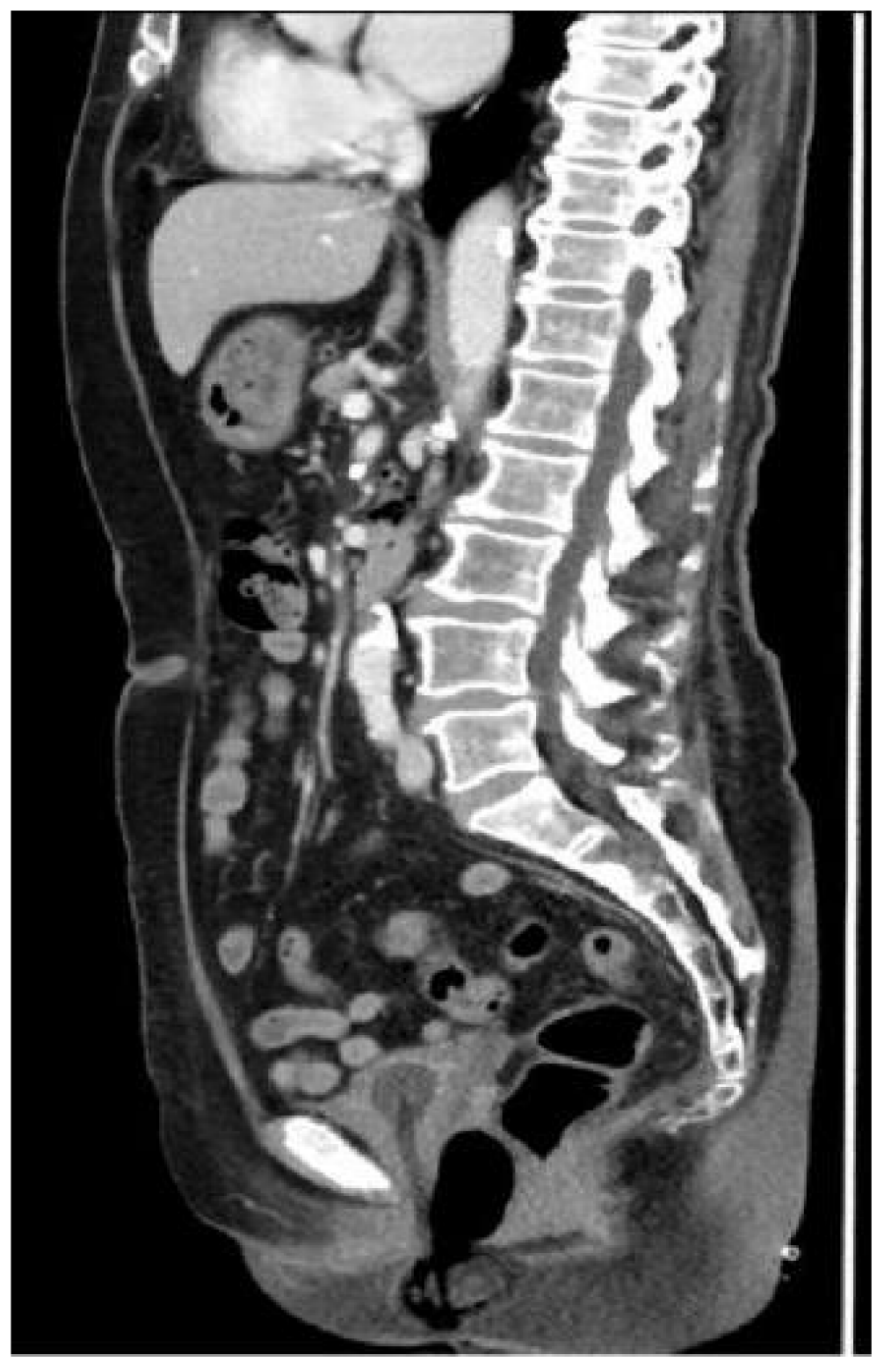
The pelvic CT scan showed that after the end of treatment, the patient achieved clinical complete remission.
